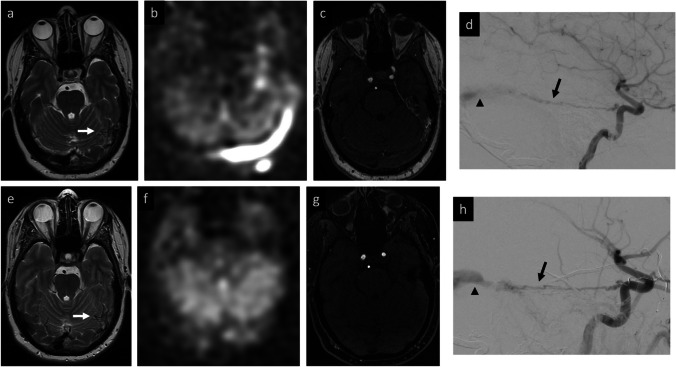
Example of ASL false negative in dAVF patient with improved but persistent AV shunting after embolization. Baseline MRI: conspicuous left tentorial flow voids (white arrow, a) with elevated ASL signal in the left transverse sinus (b) and correlative flow-related signal on 3D TOF MRA (c) compatible with shunting. Baseline DSA: left tentorial arterial feeder from ICA (black arrow) with early venous drainage (black arrowhead) (d). Post-embolization MRI: after embolization of two ECA feeders, improved but persistent flow-voids near left tentorium (white arrow, e), though without elevated ASL signal (f) or abnormal flow-related signal on 3D TOF (g) to suggest residual shunting. Post-treatment DSA: lateral projection shows the persistent, non-targeted left tentorial artery feeder (black arrow) and venous drainage (black arrowhead) compatible with residual dAVF (h)
